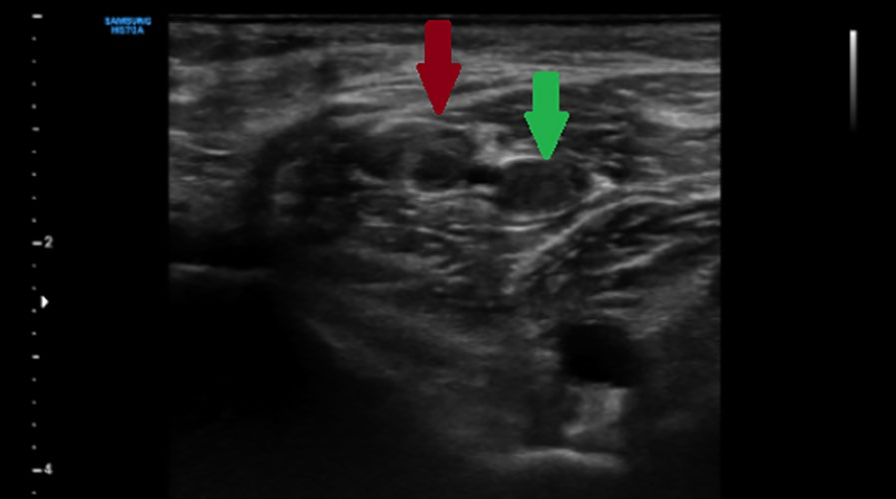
Absence of collapse after compression with the ultrasound transducer. With the transducer in the same position as Fig. 1, pressure is exerted. In the absence of thrombosis, the veins should collapse. In this case, they not collapse (arrows). This maneuver helps to confirm thrombosis of the right side vein (green arrow)
